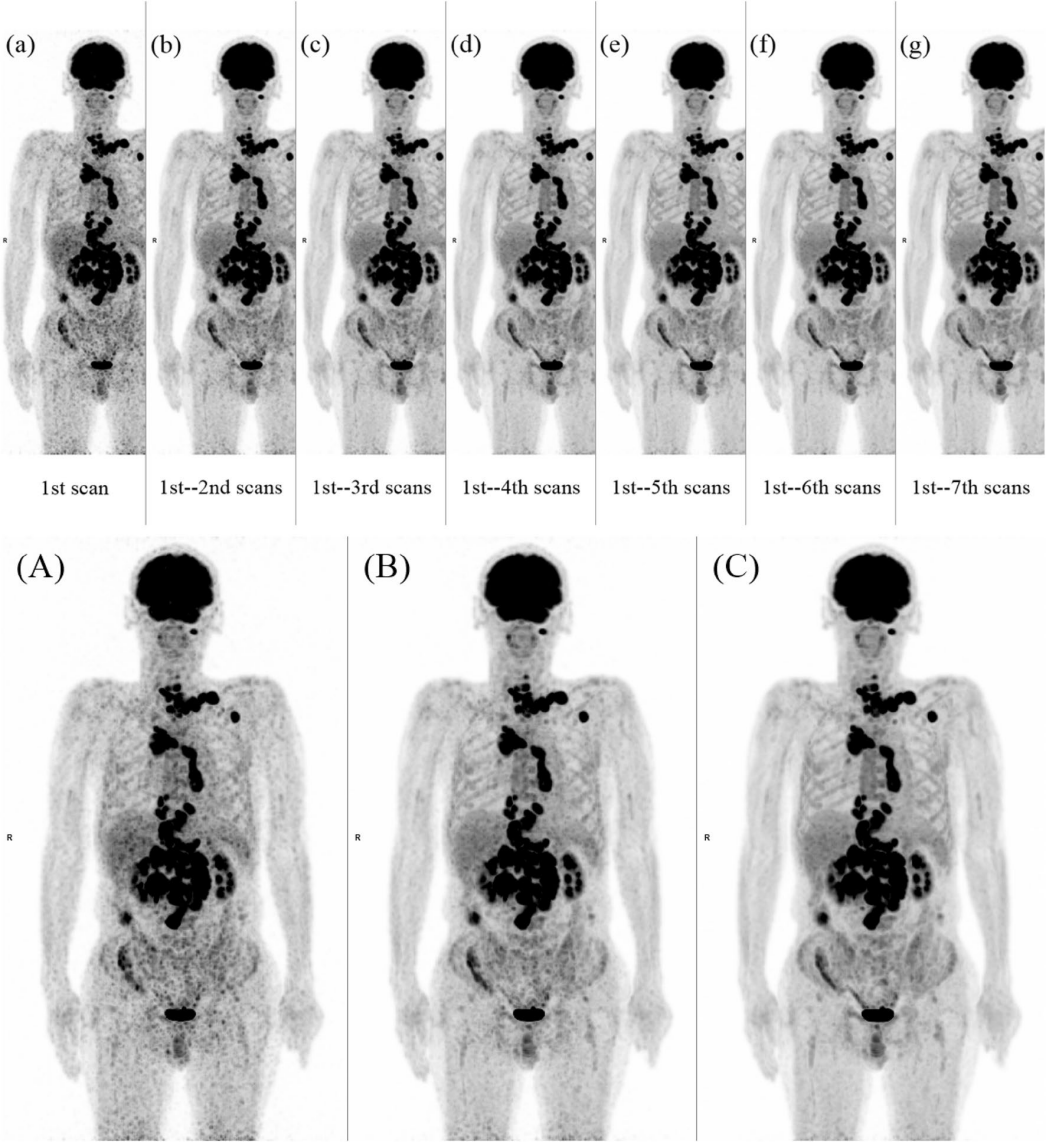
One serial whole-body dynamic ^18^F-FDG PET image a and six summed images b–g of a pretreatment malignant lymphoma patient. The image quality improves as the number of the summed images increases. Images A–C were enlarged image a, b, d as samples of quality score 1, 2 and 3
